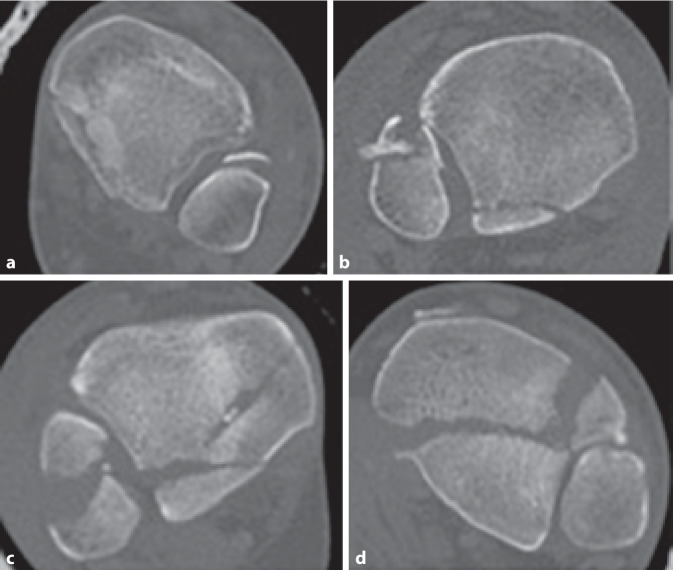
Primär erfolgten präoperative Röntgenaufnahmen des OSG (a.-p., Mortise und lateraler Strahlengang) sowie im Anschluss eine erweiterte Bildgebung mittels CT des Sprunggelenks mit axialer, sagittaler und koronarer Rekonstruktion. Die Schichtdicke belief sich auf 1–2 mm. In 64,5 % der Fälle konnten zudem 3D-Rekonstruktionen erstellt werden. Anhand der Röntgenbilder erfolgten eine Auswertung und Klassifikation der Fibulafraktur, einer etwaigen (Sub‑)Luxationsstellung sowie der Art der medialen Verletzung (Fraktur, ligamentäre Verletzung). CT und 3D-CT wurden bezüglich der Größe und Form des posterioren tibialen Kantenfragments ausgewertet. Ein den gesamten Innenknöchel einschließender Frakturverlauf wurde als Pilon-tibiale-Fraktur definiert und aus der Studie ausgeschlossen.
